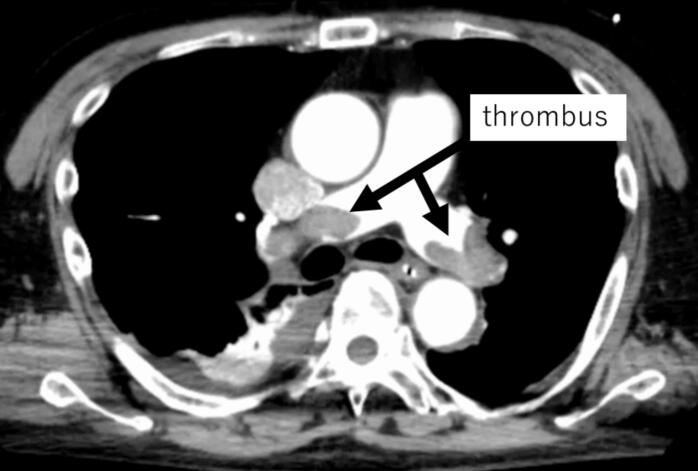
Contrast-enhanced computed tomography revealed embolisms in the main trunks of both pulmonary arteries.
